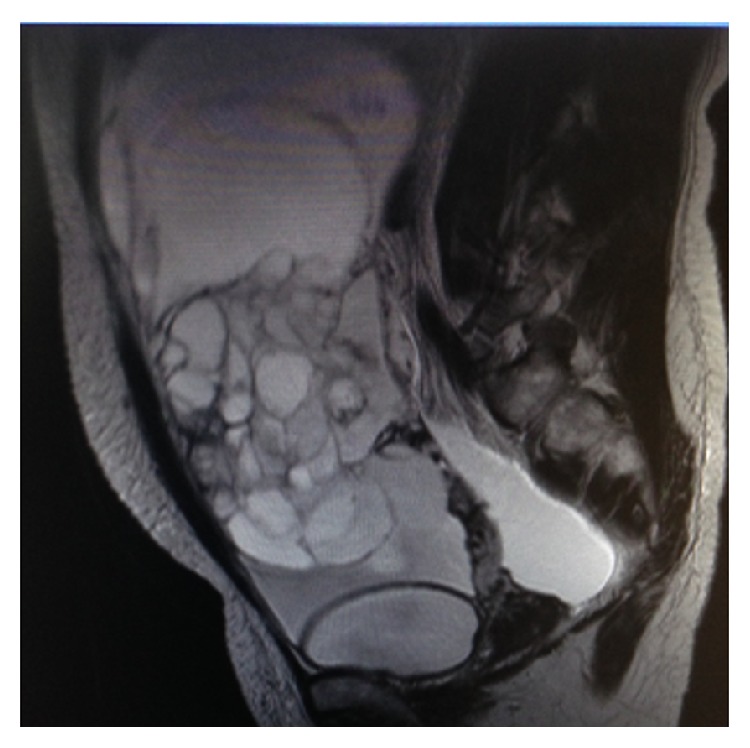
MRI image of patient 2 before operation.
